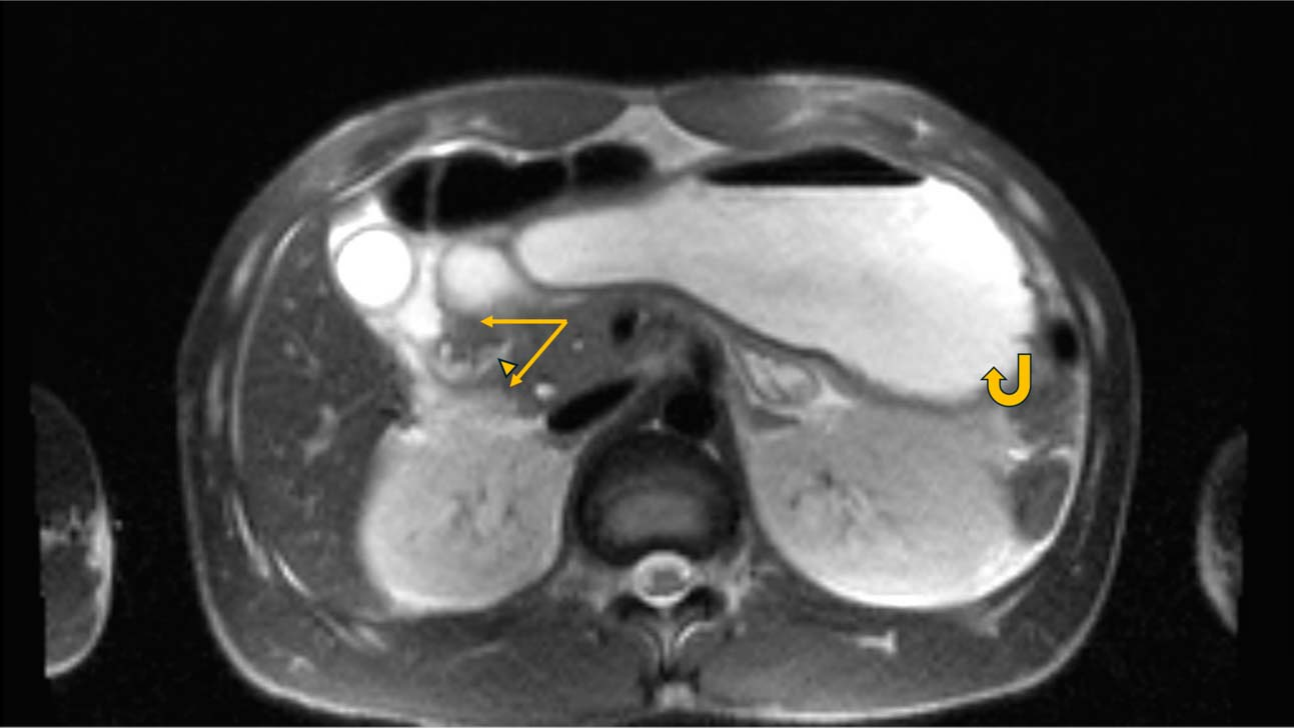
Axial T1 magnetic resonance imaging depicting a complete annular pancreas (arrows) around the proximal second portion of the duodenum(arrowhead), with an enlarged stomach (curved arrow).
